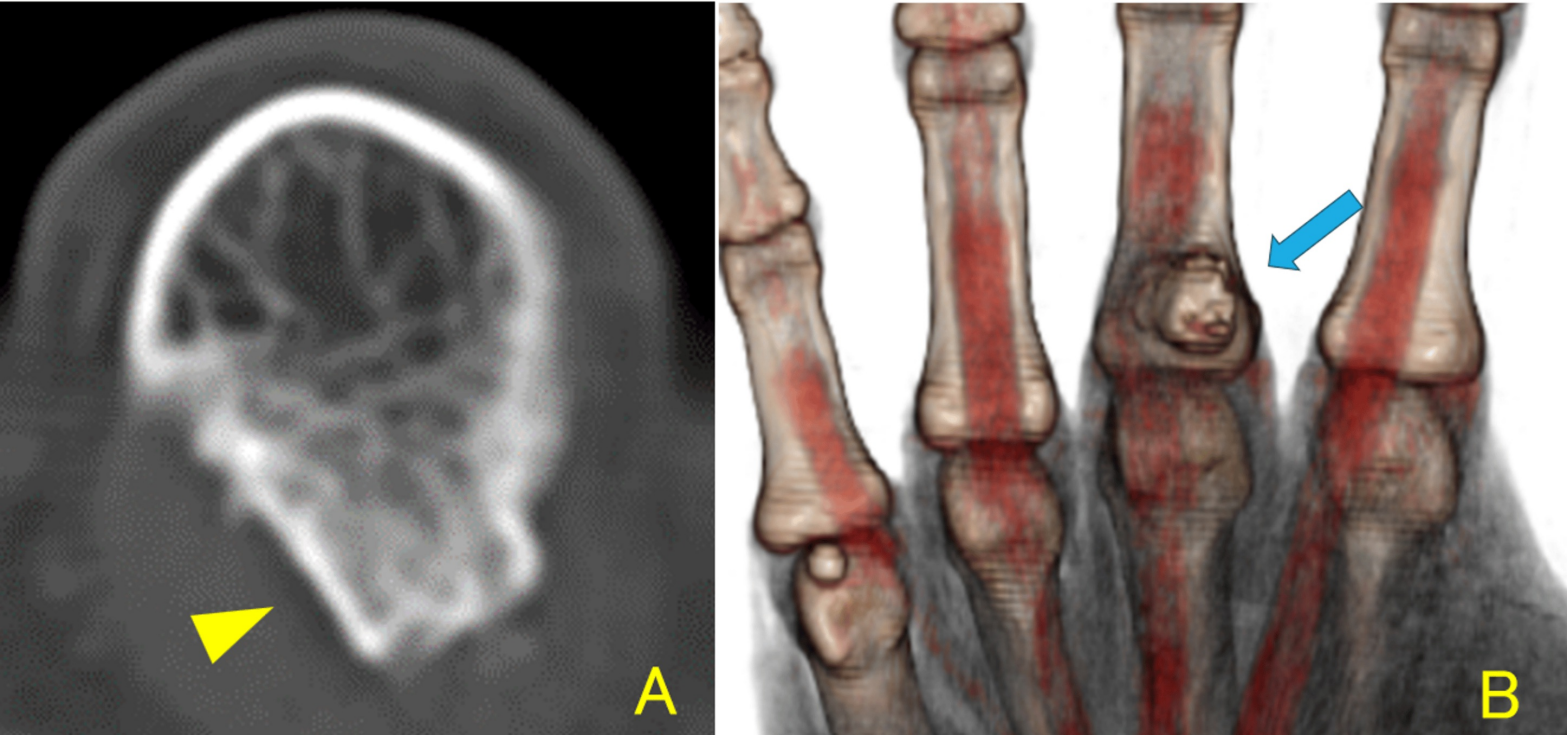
Computed tomography. (A) A 13 × 10 × 9 mm-sized bony prominence in continuity with the bone marrow is visible. (B) Ulnar deviation of the flexor tendon is visible.
